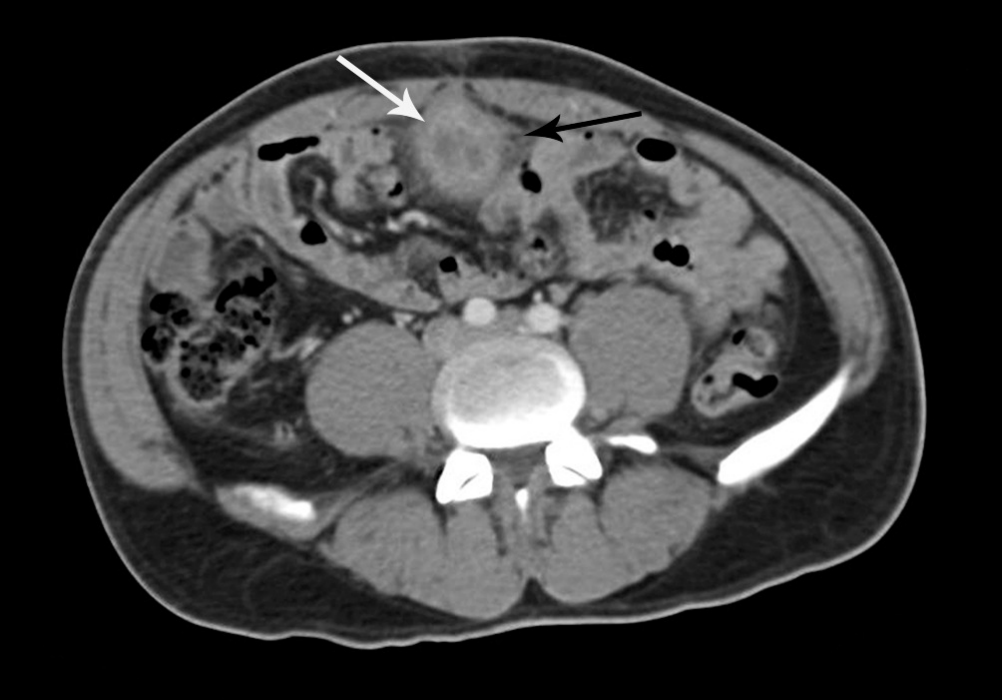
Axial CT image showing abscess formation in patent urachus posterior to the abdominal muscles. The rim enhancement with contrast (white arrow) and the fat stranding (black arrow) are significant.
